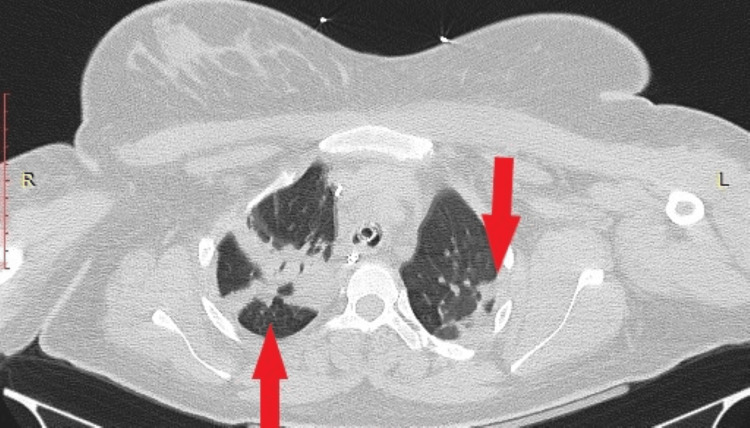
Computed tomography (CT) of chest in the axial plane with intravenous contrast showing atelectasis of both lower lobes.
